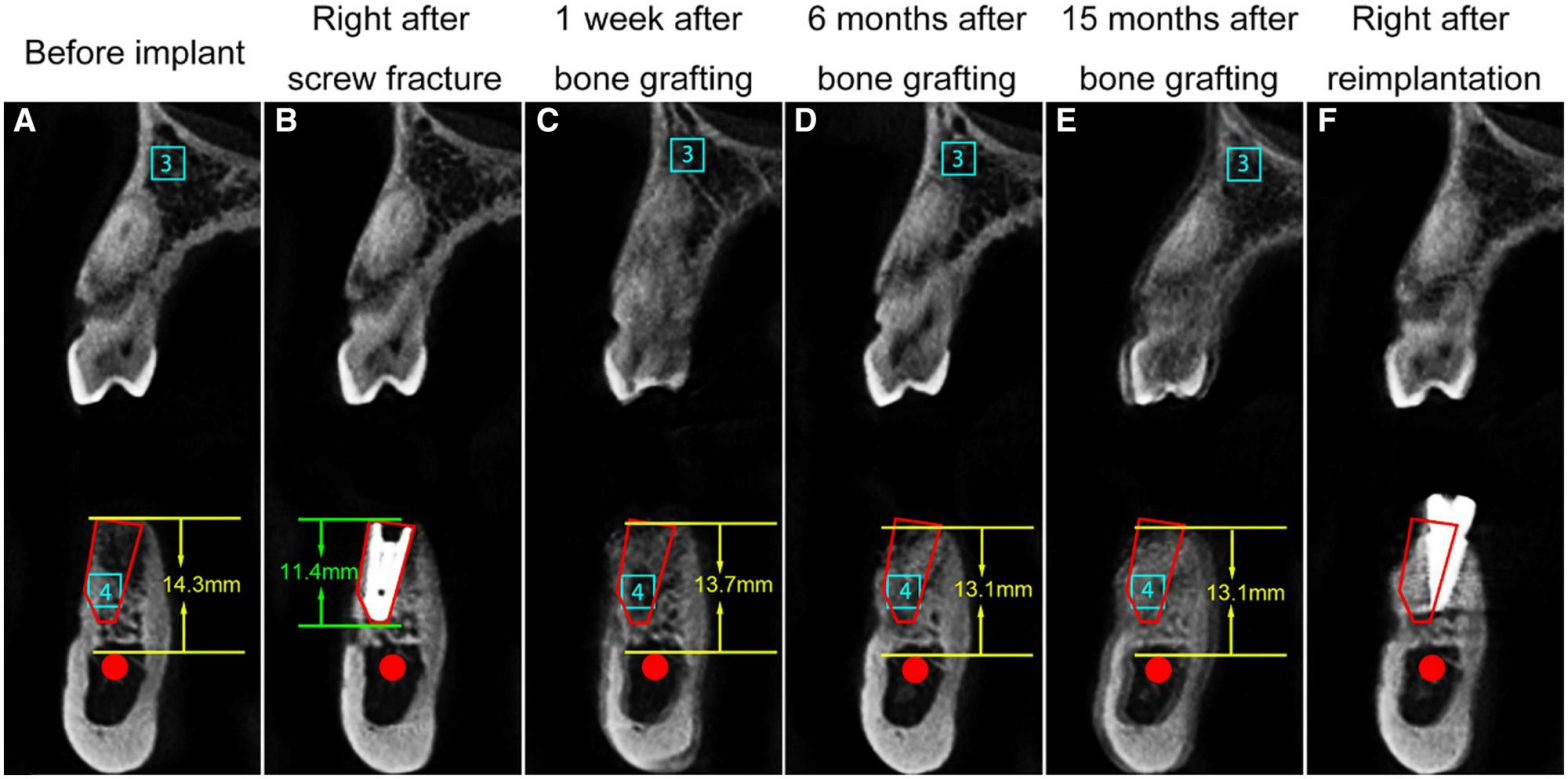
Coronal CBCT sections at different time points: (A) before implant, (B) immediately after screw fracture, (C) 1 week after bone grafting, (D) 6 months after bone grafting, (E) 15 months after bone grafting and (F) immediately after reimplantation. The red box represents the bone defect, and the red dot indicates the mandibular nerve canal.
